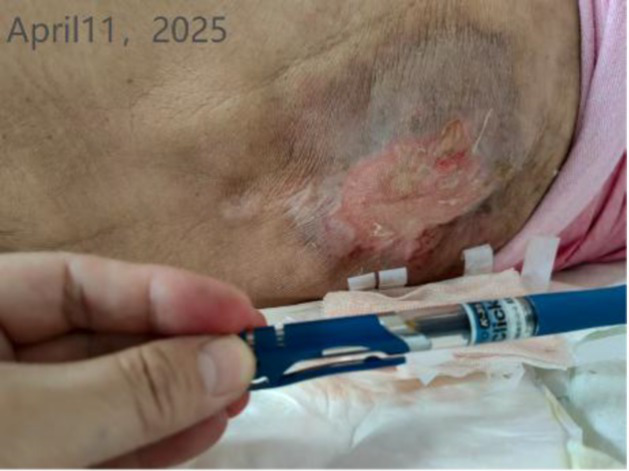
Detailed evaluation: Wound location: Thoracolumbar junction of the spine; dimensions: 10 cm × 5 cm × 0 cm; Tissue composition: Red tissue 60%, white tissue 30%, epithelialized tissue 10%. Pigmentation is present around the ulcer. Local manifestations: Increased pain, swelling at the upper right of the wound (10 cm × 8 cm × 1 cm), soft texture with fluctuance, slightly elevated skin temperature; moderate exudate, pink/red in color, odorless; NRS pain score of 3. General condition: Fever, elevated C-reactive protein, decreased albumin (hypoalbuminemia). (continued in the next column)	Diagnostic and therapeutic reflections: Analysis of the Biomechanical Mechanism Behind Recurrent Pressure Injuries: Patients with syringomyelia experience impaired voluntary movement, and prolonged bed rest leads to persistent pressure at the thoracolumbar junction of the spine. This constitutes the core factor driving recurrent pressure injuries. Evaluation and Adjustment of Preventive Measures: Although an air-filled mattress is the standard protocol for preventing pressure injuries, the patient’s family expressed concerns about its potential adverse effects on syringomyelia. Following the discussion, its use was temporarily discontinued. Instead, pressure distribution will be enhanced by increasing repositioning frequency and utilizing soft padding support. Continuity Considerations for Local Treatment Protocol: Given the patient’s prior successful treatment of pressure injuries with our hospital’s Jin Di Sheng Ji Ointment, this regimen will be maintained to ensure therapeutic continuity. Diagnosis: Stage 2 pressure injury Action plan: Wound Care: After disinfecting with povidone-iodine, wipe with saline and allow to dry. Apply Jin Di Sheng Ji ointment externally (once daily). Systemic Support: Enhance nutrition with intravenous infusion of 10 g albumin. Nursing Emphasis: Timely repositioning, Soft cushion support for pressure relief, Management of urinary incontinence (high-absorbency care products), and maintaining local cleanliness and dryness. Recommendation: Perform a color Doppler ultrasound examination of the subcutaneous swelling area. If necessary, consult with a spinal surgeon. The patient’s family has been informed of the condition’s progression and the proposed treatment plan. They have expressed understanding and will cooperate with the treatment. Close monitoring of ulcer exudate and surrounding skin changes is required. Should signs of infection emerge—such as increased redness, swelling, heat, or pain, or elevated body temperature—a complete blood count and wound exudate analysis should be promptly conducted. Antibiotic regimen adjustments may be necessary. Concurrently, the patient’s overall condition must be closely observed to maintain fluid and electrolyte balance and prevent complications.
